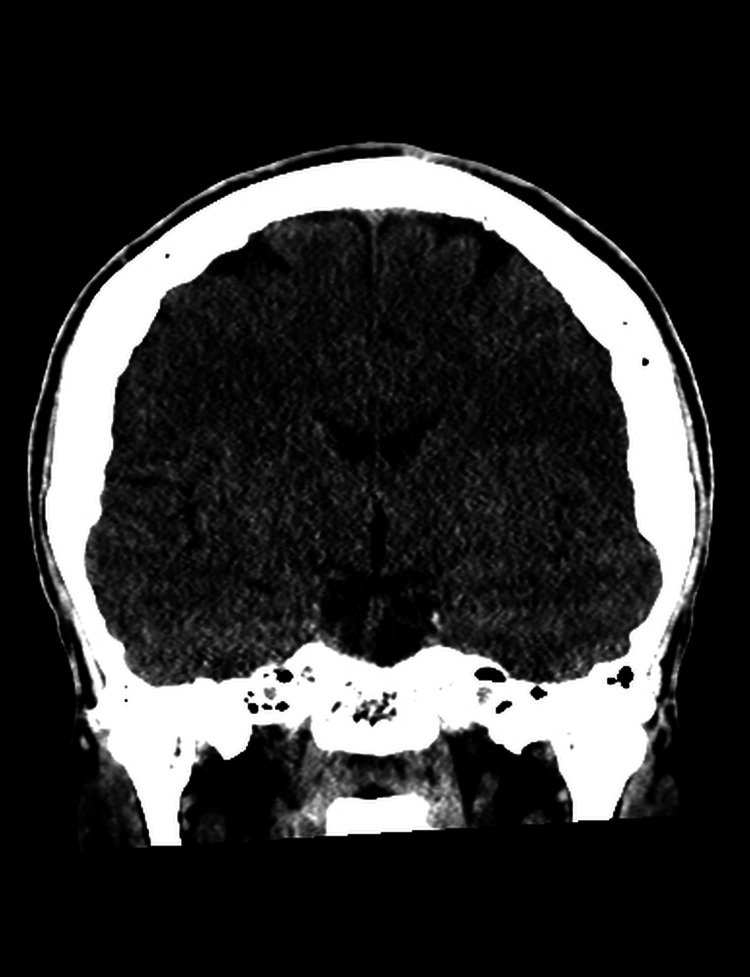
Noncontrast CT of the brain (coronal view). The image demonstrates no acute pathology involving the basal ganglia, thalami, or temporal lobes. No mass lesions, hemorrhage, or focal areas of hypoattenuation are identified. The findings are consistent with the absence of opportunistic infection or other organic etiology accounting for the patient’s acute neuropsychiatric presentation.
